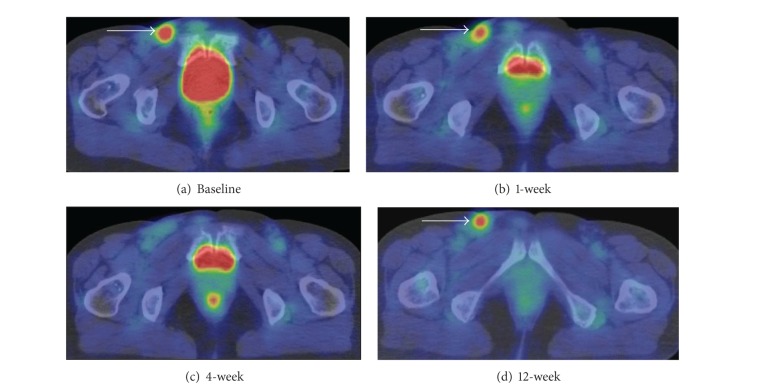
Axial images at level of inguinal region of patient 7. (a) Baseline, (b) 1-week scan, (c) 4-week scan, and (d) 12-week scan. FDG-avid lesion is seen in the right inguinal lesion (arrow). FDG uptake decrease for up to 4 weeks and the degree of the uptake is not remarkable at 4 weeks, but the declining metabolism turns to increase from 4 weeks to 12 weeks. Physiological activities are seen in the bladder and the rectum.
